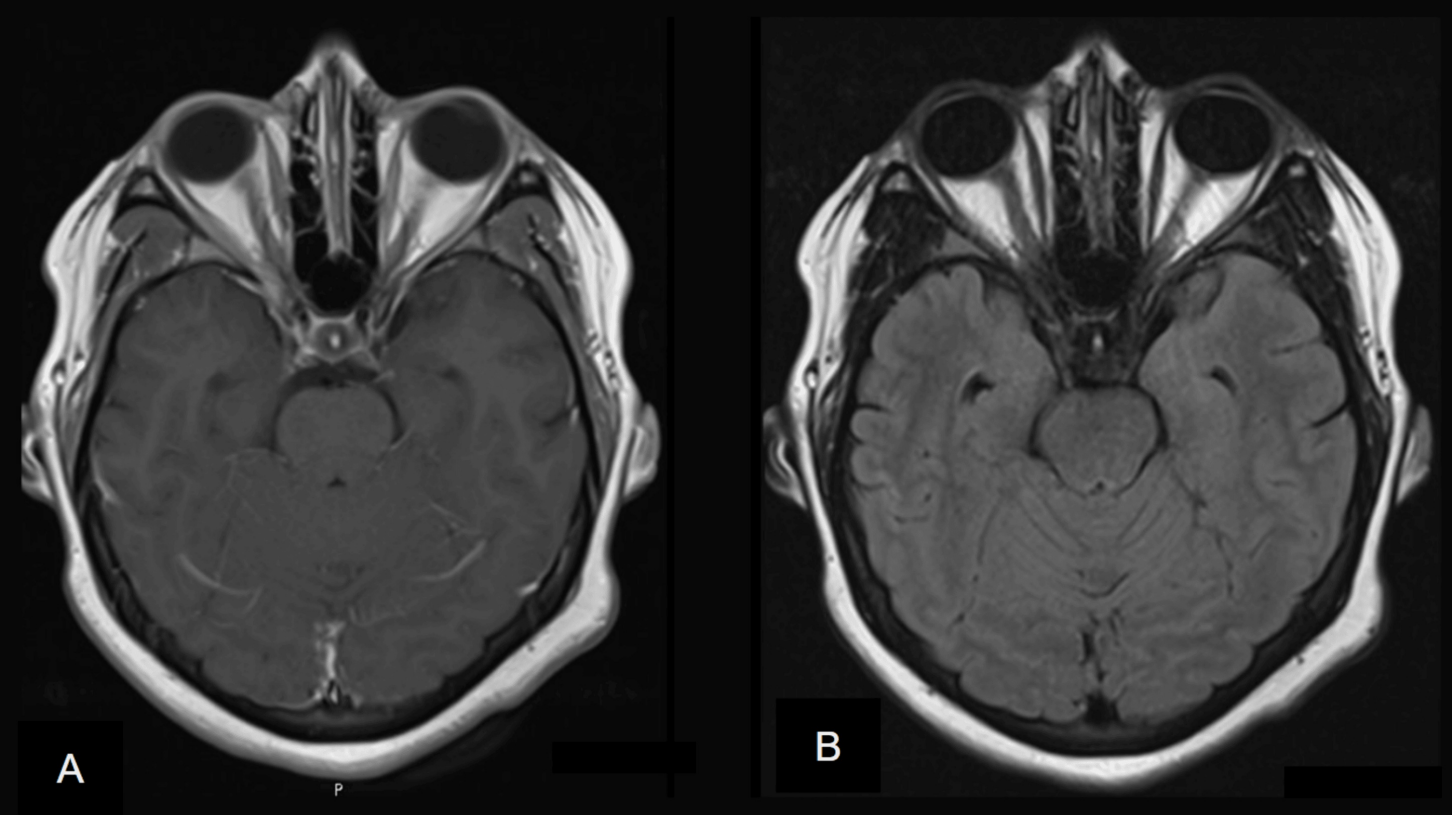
A) T1-weighted MRI post-gadolinium injection and B) Axial FLAIR MRI with normal-appearing optic nerves, extraocular muscles, brain parenchyma, and pons. FLAIR MRI: Fluid-attenuated inversion recovery magnetic resonance imaging
